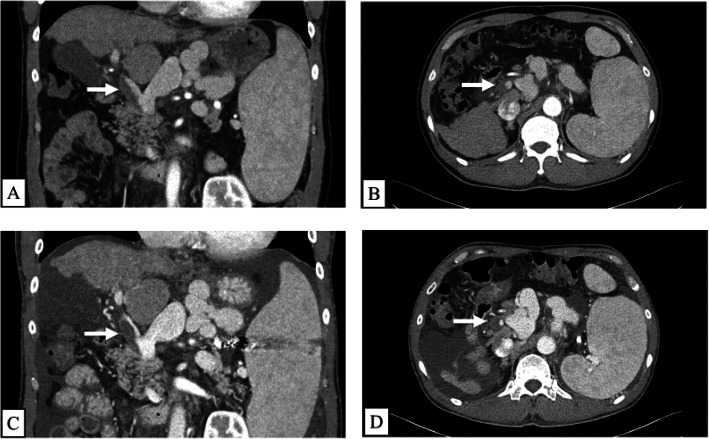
The CT findings of the pre-transplant PVT before and after the administration of AT III. The Yerdel classification of the pre-transplant PVT was grade II before the administration AT III (a and b). However, the pre-transplant PVT was progressed regardless of the administration AT III (c and d)
